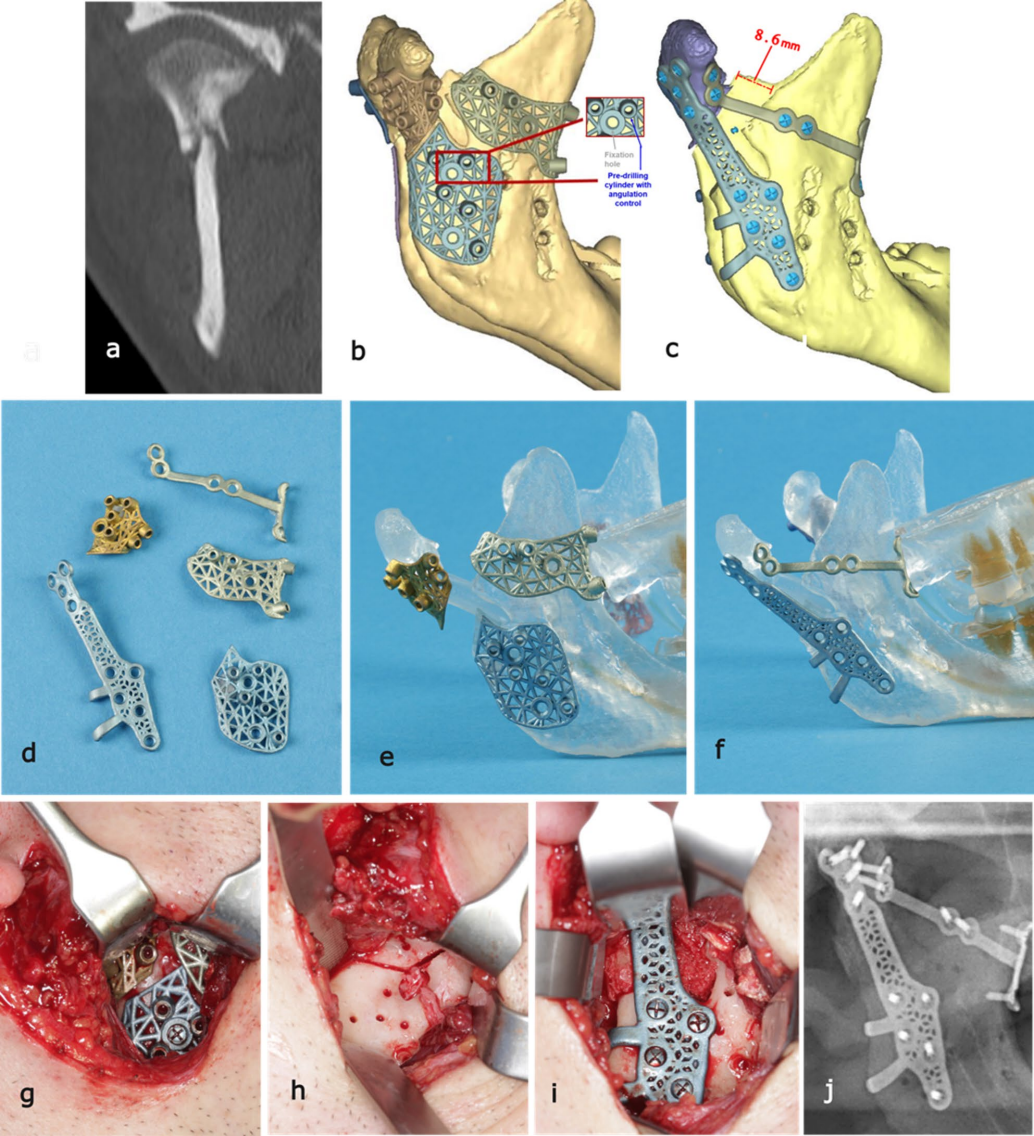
Demonstration of case 1, the most complex case in this series: a CT-scan showing a non-union with loss of ramus height in the right condyle after a base fracture. b Planned position of the patient-specific osteotomy and fixation guides. c Planned position of the patient-specific fixation implants. d-f Patient-specific armamentarium, including cutting/pre-drilling guides and fixation implants, displayed in their planned positions. g-i Intraoperative photographs showing, respectively, the cutting/pre-drilling guides in place, the completed osteotomy with pre-drilling, and the main PSI after fixation and transplantation of the iliac crest bone. j Postoperative panoramic radiograph showing the final position of the patient-specific implants.
